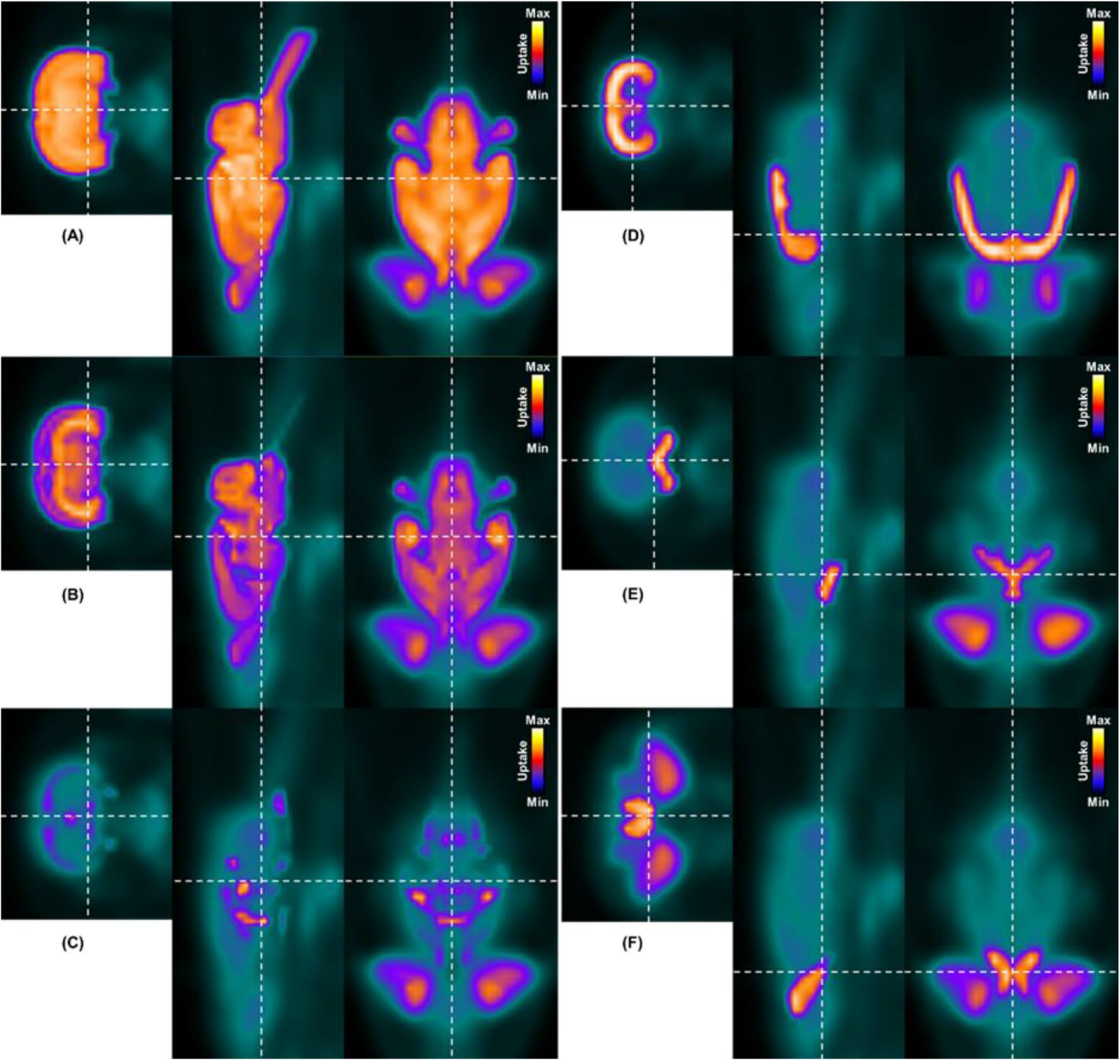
Co-registered PET image template based on the sham-operated controls as well as following anatomical image information: MRI T2* image (A), gray matter (B), white matter (C), the neocortex (D), the basal forebrain region (E) as well as the olfactory system (F).
